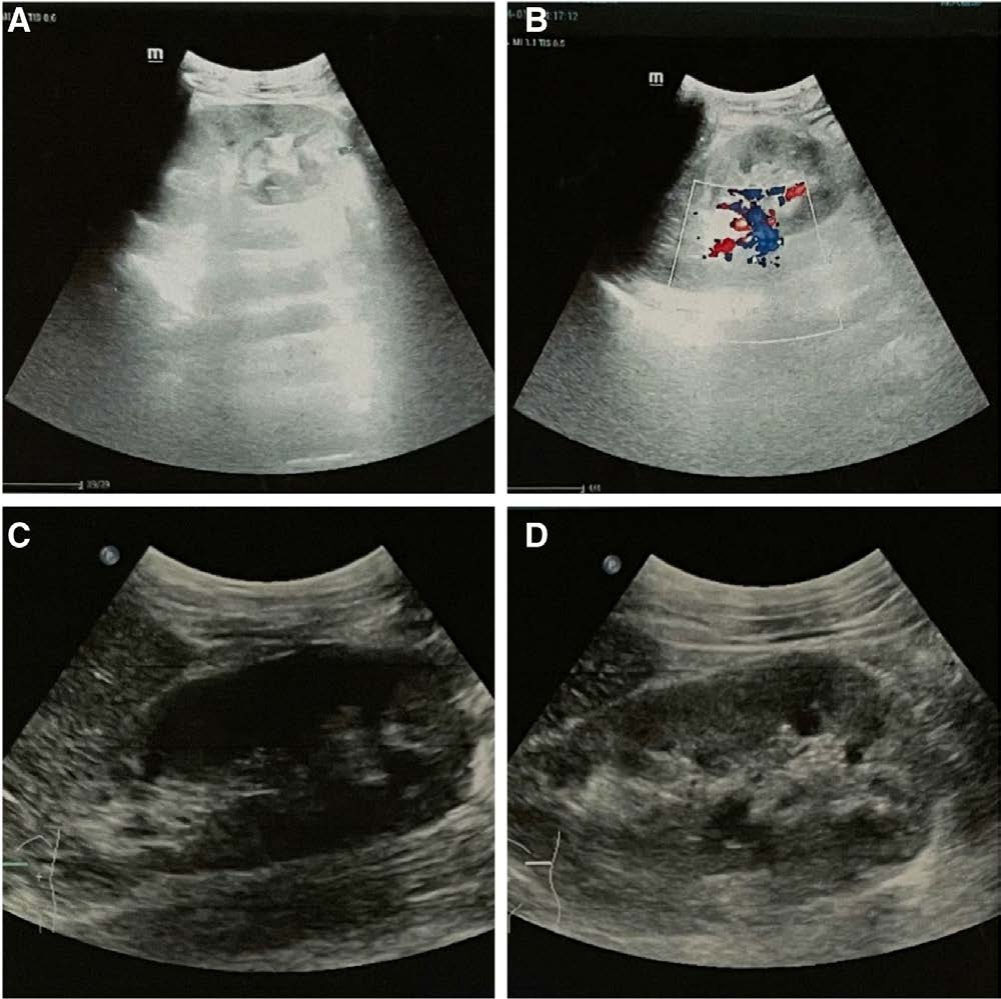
(A) (B) On admission, abdominal ultrasound showed the shape and size of both kidneys were normal, along with clear demarcation of renal cortex and medulla without hydronephrosis and abnormal echoes. (C) (D) Abdominal ultrasound showed no abnormalities in both kidneys after 6 courses of cyclophosphamide.
